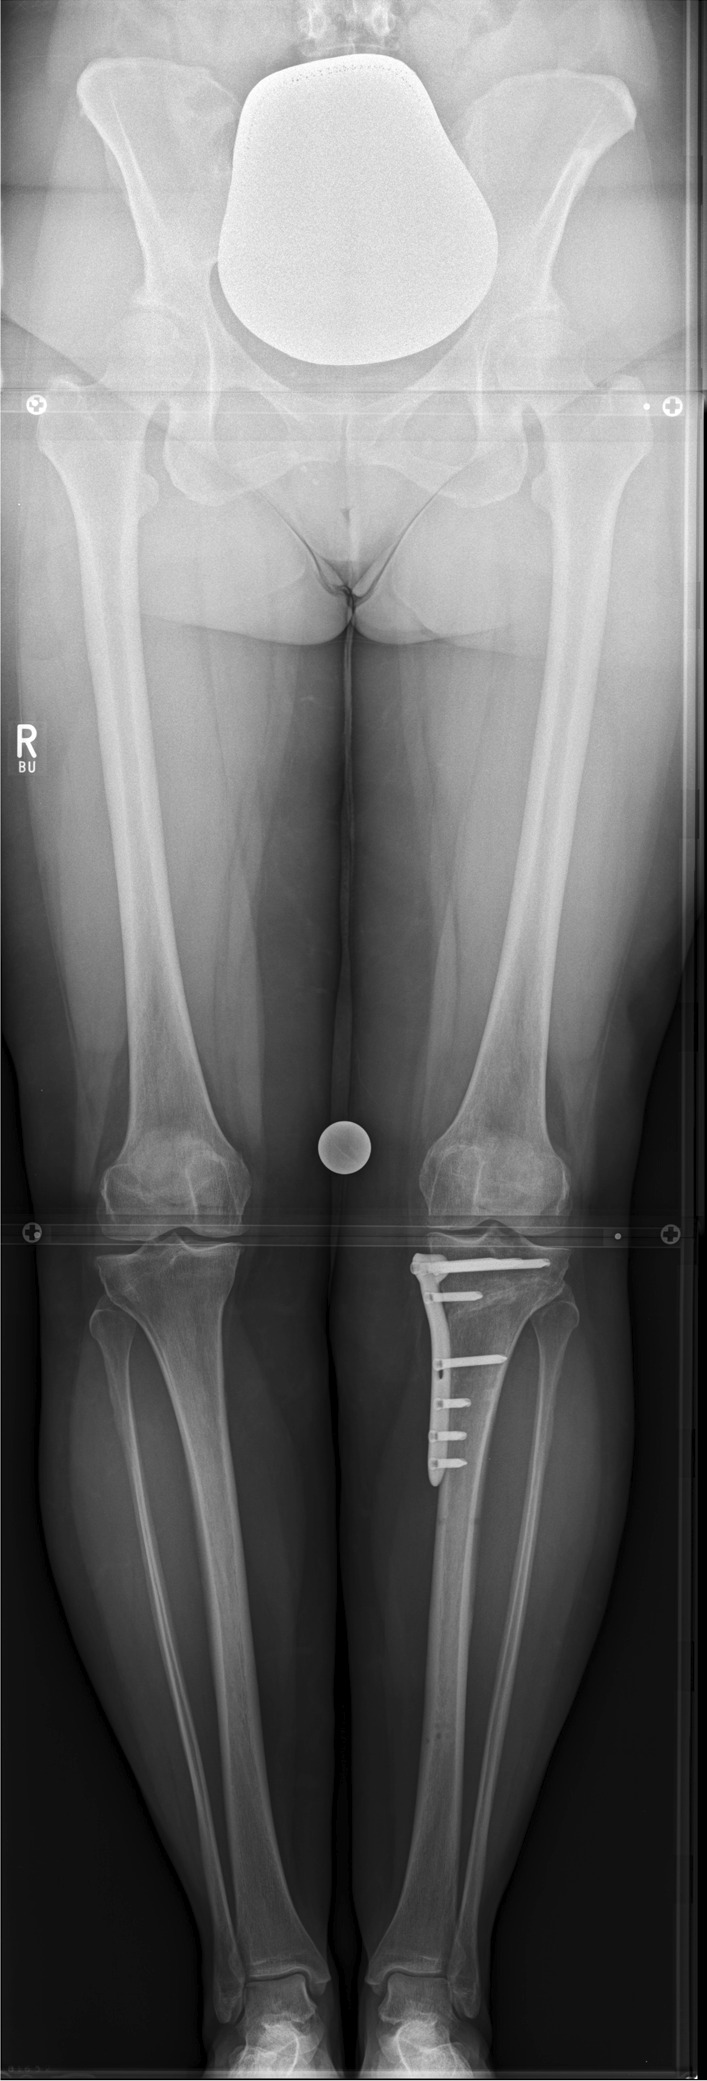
Long-leg standing radiograph showing normal knee alignment and increased ischiofemoral space postoperatively (after closed wedge high tibial osteotomy)
